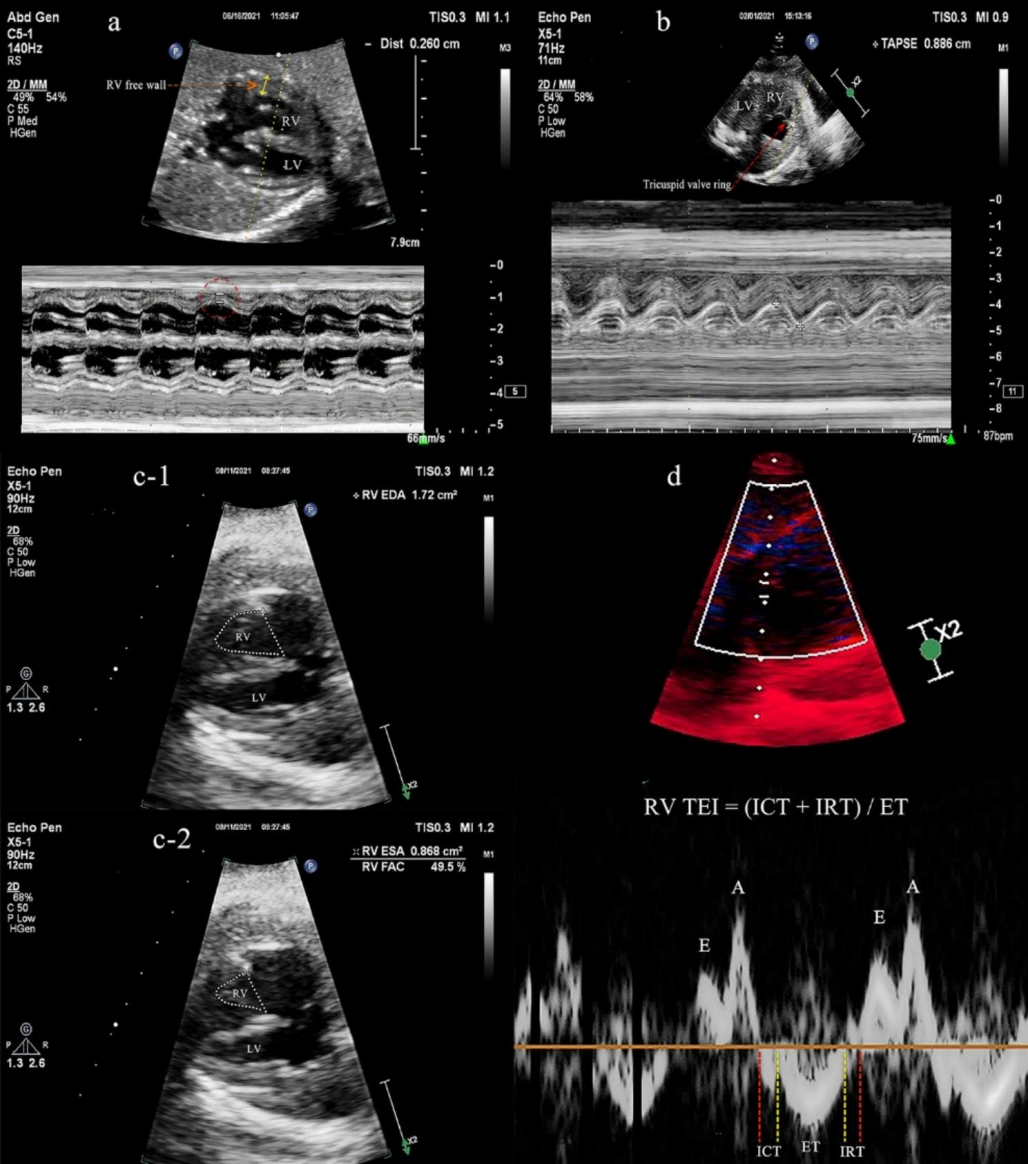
Conventional fetal echocardiography (a) Right ventricular free wall thickness; (b) Tricuspid annular plane systolic excursion, longitudinal displacement of the tricuspid annulus from end systolic to end diastolic measured on the obtained tricuspid annulus motion curve; c-1-2. Right ventricular fractional area changes, (end-diastolic area - end-systolic area)/end-diastolic area ×100%; d. Right ventricular Tei index (RV TEI = (ICT + IRT)/ET, *ICT, isovolumetric contraction time; IRT, isovolumetric relaxation time;* ET, ejection time)
